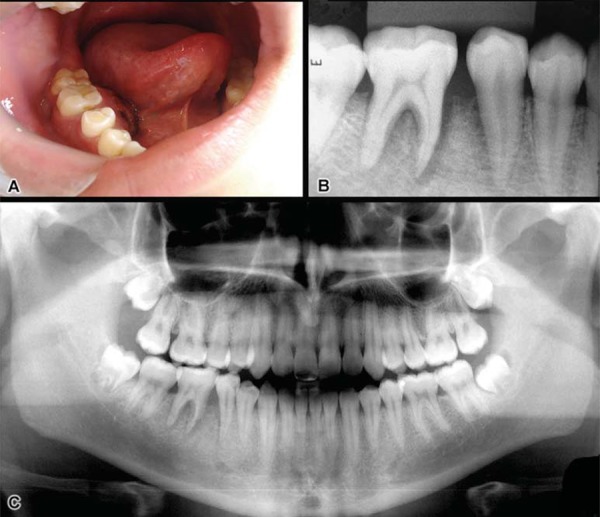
Clinical aspect (A) showing expansion of buccal and lingual cortical plates of the right mandible in the region of teeth #29, #30, and #31, with some redness and apparently intact overlying mucosa. Periapical and panoramic radiographs (B-C) showing slight apical radiolucency in the roots of teeth #29 and #30 with loss of lamina dura and periodontal ligament space widening
